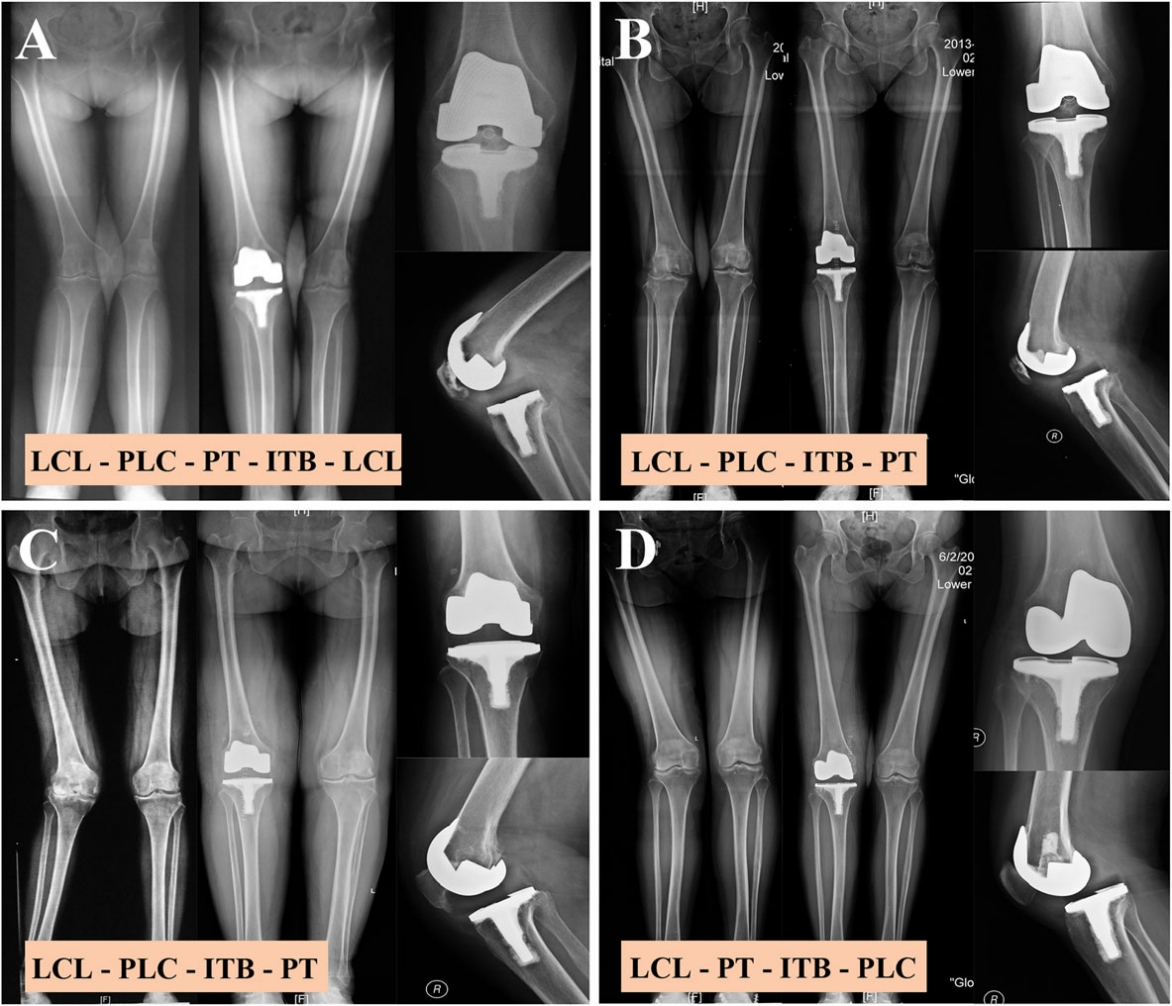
Radiographs of the dislocated patients showing the structures released and the release sequence. a, b, c, and d represent patients 1, 16, 29, and 34 in Table 1, respectively. The release sequence was shown for each patient. ITB stands for the iliotibial band; PLC, posterolateral capsule; PT, popliteal tendon; LCL, lateral collateral ligament
